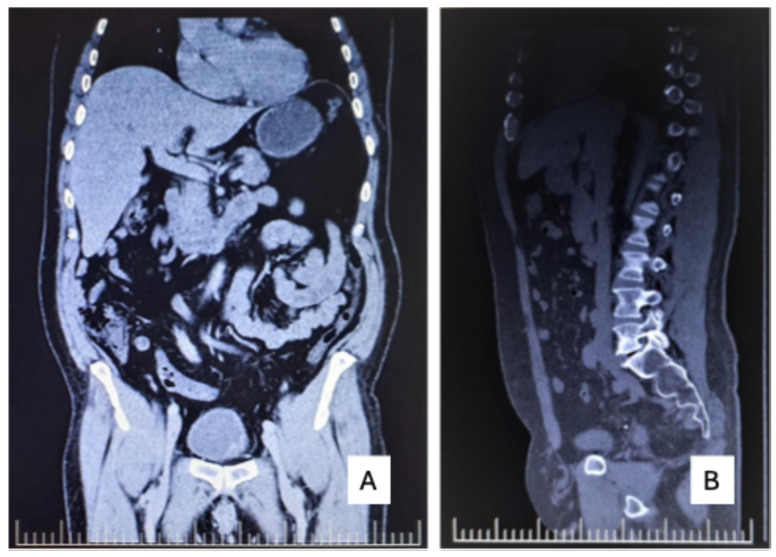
Contrast-enhanced Uro-CT demonstrating bladder wall abnormalities. (A) Coronal contrast-enhanced CT reconstruction showing focal thickening and enhancement of the left bladder wall, suspicious for urothelial pathology. (B) Sagittal reconstruction confirming focal bladder wall thickening. A non-obstructive right iliac ureteral calculus is additionally noted.
